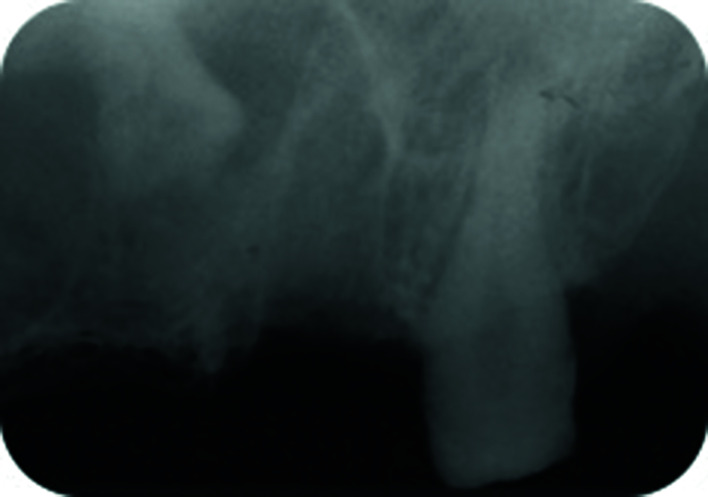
Periapical x-ray view showing the fragment of the palatal root of 2.7 (R) displaced into the left maxillary sinus.
